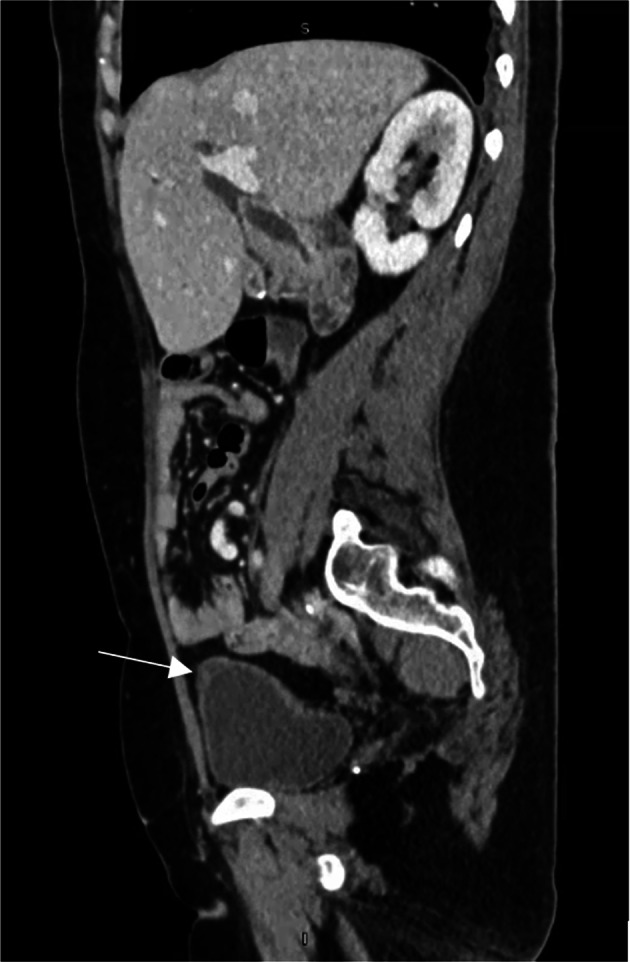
Sagittal view of non-calcified urachal cystic tumor as seen on CT imaging roughly 2 weeks pre-operative noted with white arrow. Tumor remained unchanged in size on imaging from 3 years prior to excision
